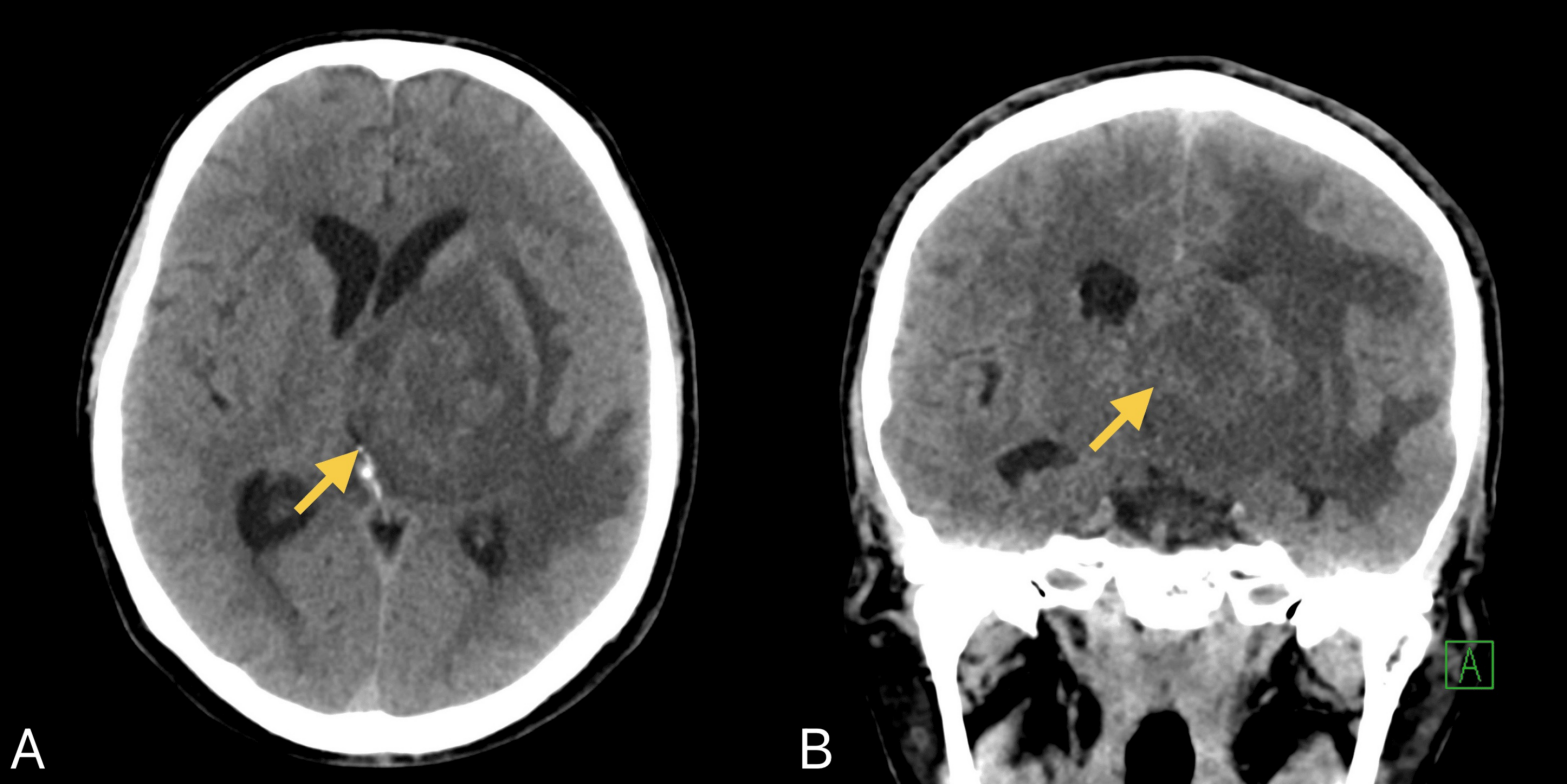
Non-enhanced CT scan of the brain in axial (A) and coronal (B) views showing a large left thalamic lesion associated with significant edema causing rightward midline shift and left uncal herniation. CT: computed tomography
